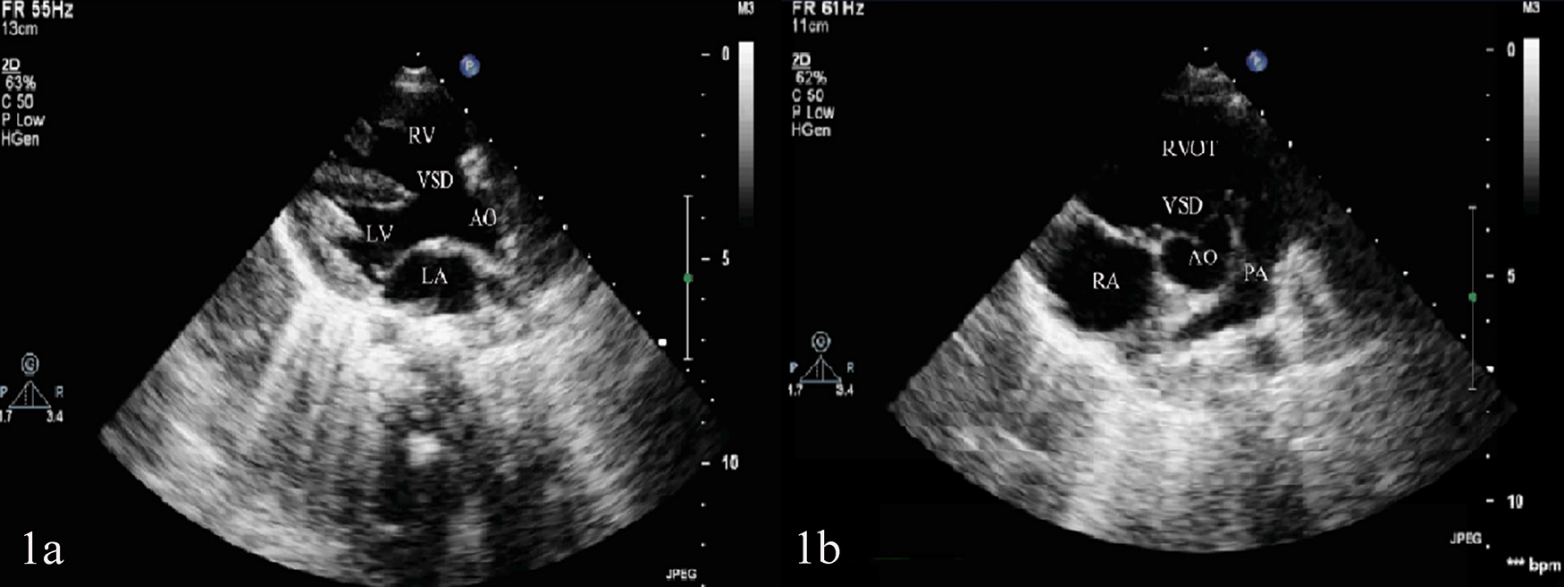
(A) Ventricular septal defect and aortic overriding shown in the left ventricular long-axis view of transthoracic echocardiography. (B) Pulmonary artery stenosis shown in the aorta short-axis view of transthoracic echocardiography. LA: left atrium; LV: left ventricle; AO: aorta; VSD: ventricular septal defect; RA: right atrium; RV: right ventricle; PA: pulmonary artery; RVOT: right ventricular outflow tract.
